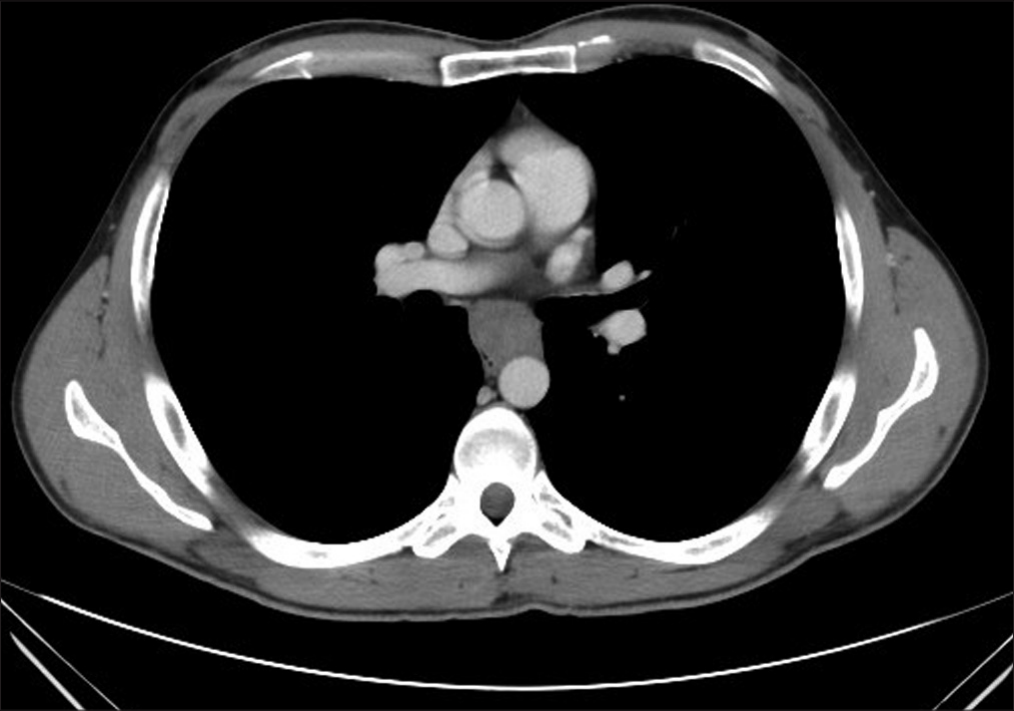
CT scan at the level of the carina showing leiomyoma
